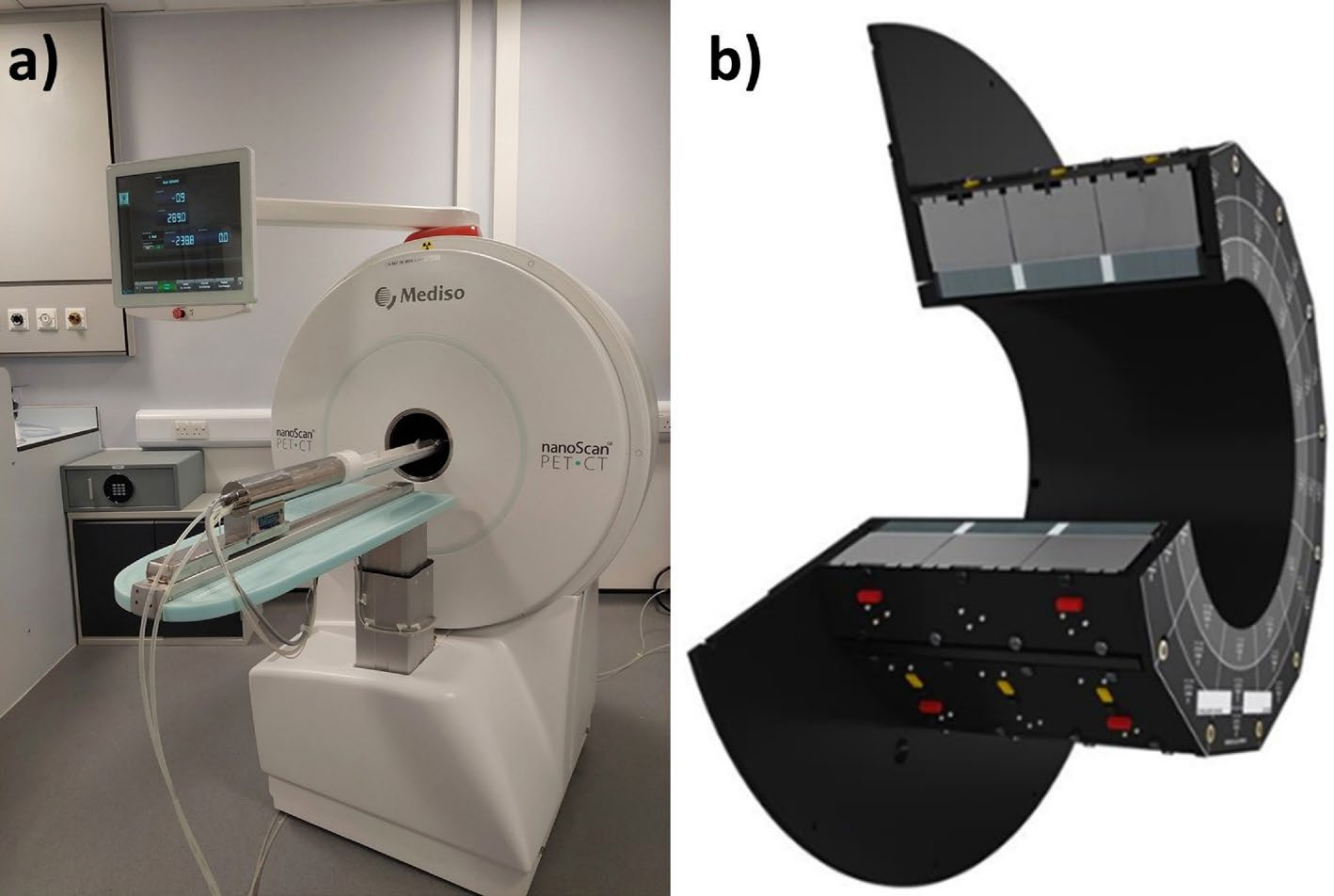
a Image of the nanoScan® P123S PET/CT system. b The extended FOV PET ring
